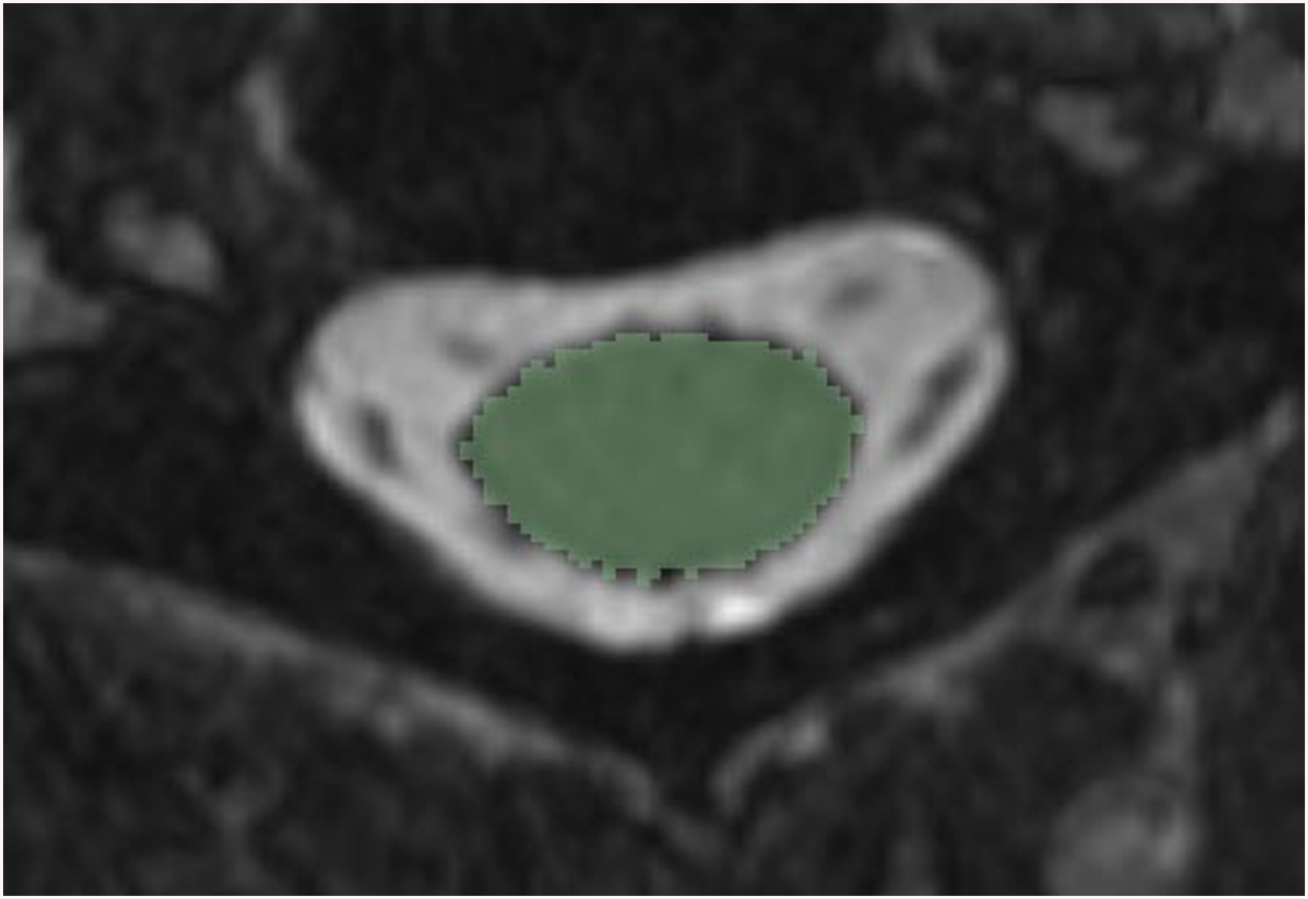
One manually segmented axial slice of a MR image. This image was shown to the raters to instruct them on how to manually segment the spinal cord.
